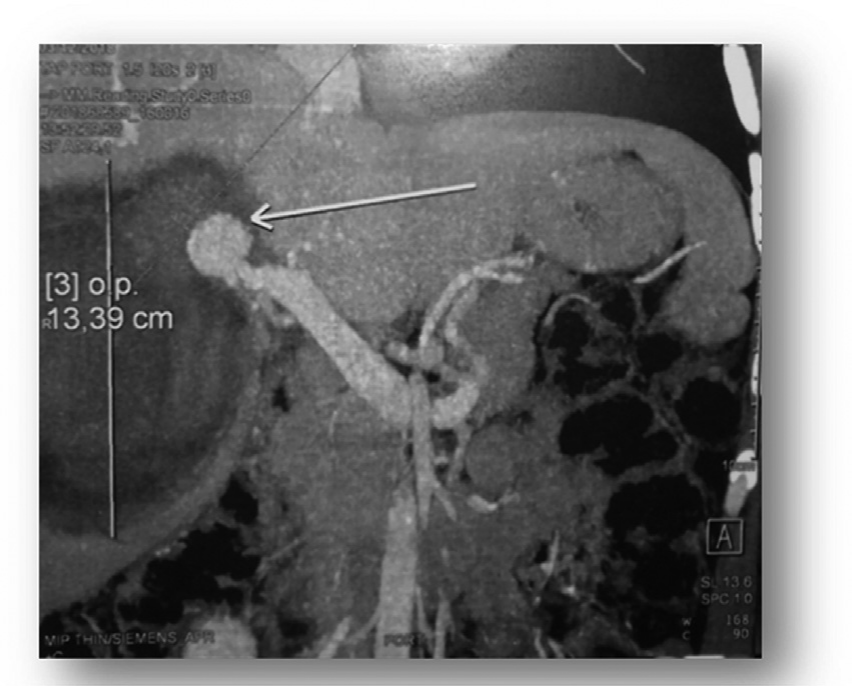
CT scan revealed an important focus of hepatic contusion occupying the IV segment of the liver with within it a spontaneously dense oval formation.
